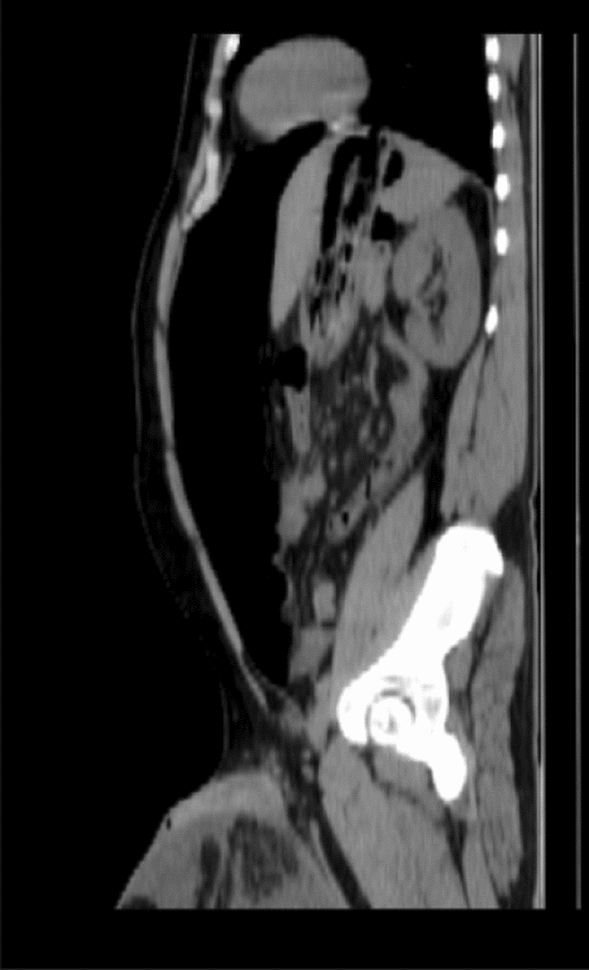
CT scan of the patient’s abdomen after PPP
